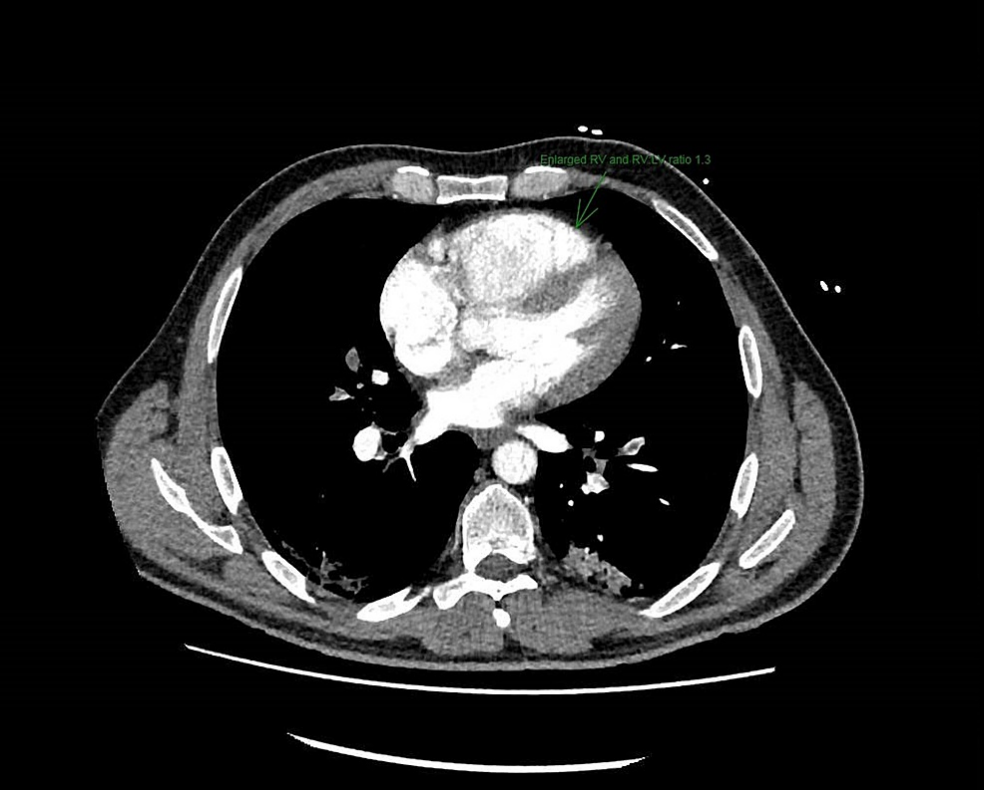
CTPA showing right ventricular strain and pulmonary embolism CTPA: computed tomography pulmonary angiography
